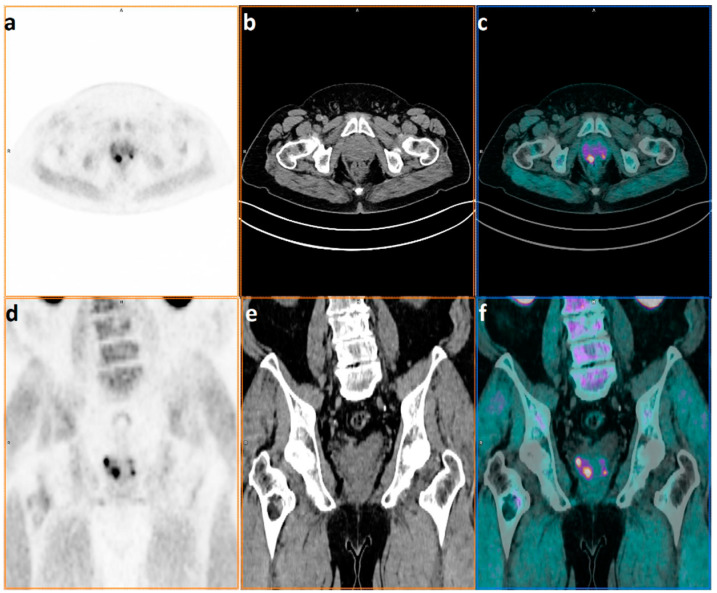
Patient with diagnosis of prostate cancer referred to ^18^F-FECh PET/CT for staging in May 2021; Gleason Score 9 = 4 + 5 and PSA value at diagnosis of 9.74 ng/mL. PET only, co-registered CT only, and hybrid PET/CT images in axial (a–c) and coronal plans (d–f) showing a multifocal pattern with four areas of focal uptake in the prostate, of which the most evident is placed in the peripheral zone of the right lobe.
